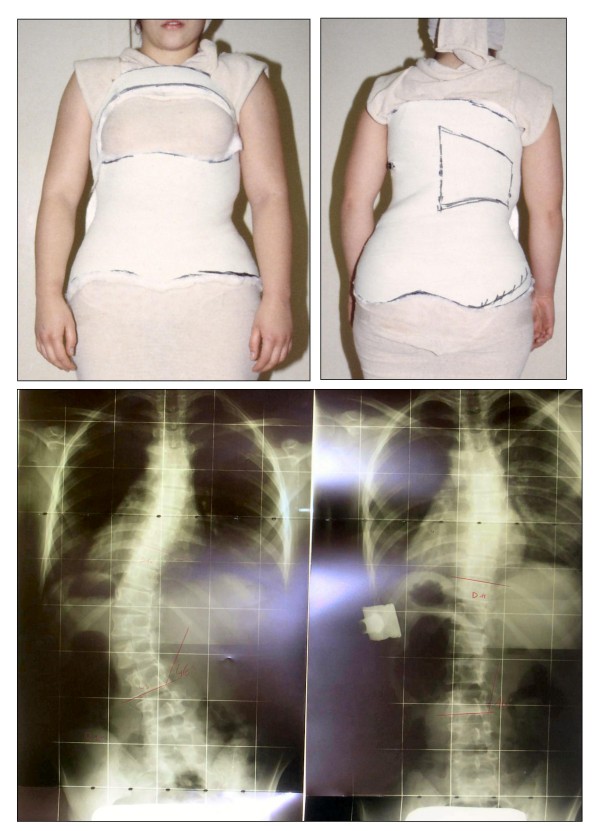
The Risser cast. The Risser cast for a thoraco-lumbar left curve, in the antero-posterior and postero-anterior views. An example of in-brace x-ray primary correction is reported.
